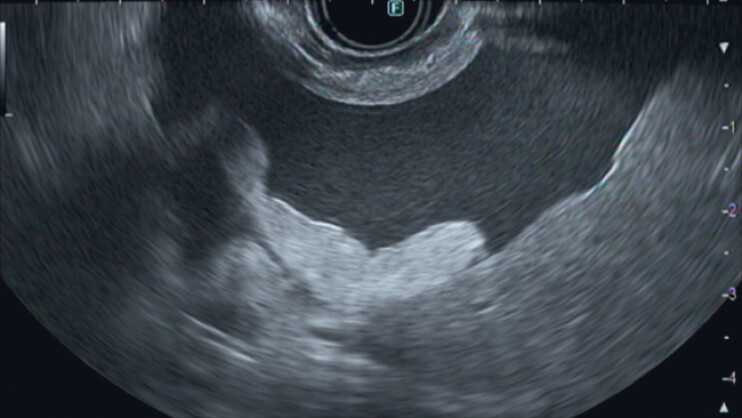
Endoscopic ultrasound images taken from the stomach showing moderate ascites between the stomach and the liver.
